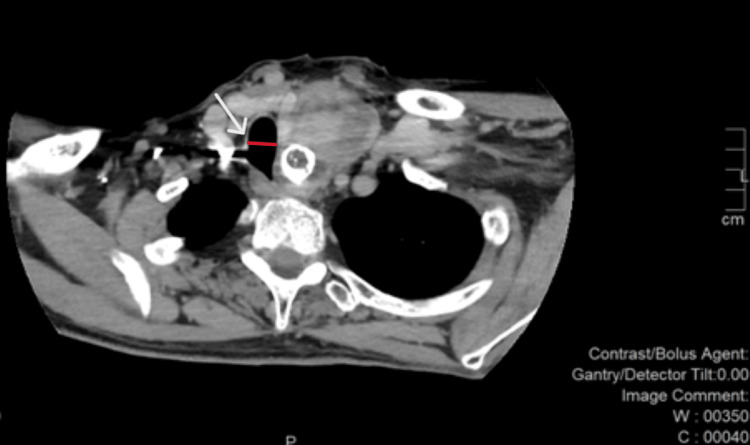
Neck CT - axial view. Tumor calcification compressing and deviating the trachea. The narrowed tracheal segment measures 1.27 cm in diameter, as indicated by the white arrow and the red line.
